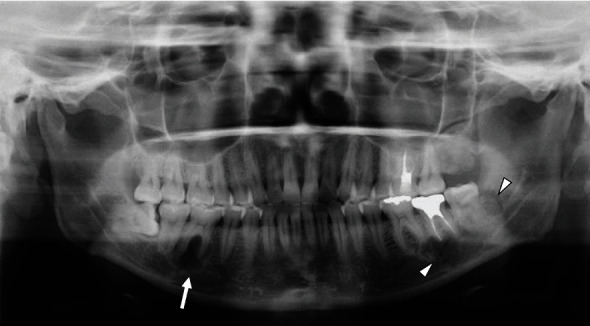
Panoramic X-ray radiogram. Radiolucent lesions are seen in the right mandible (*arrow*). The high-density lesion is complicated by the periapical right mandibular 2nd molar. Additionally, mixed radiolucent/radiopaque lesion was shown in the left molar region (*arrowheads*).
